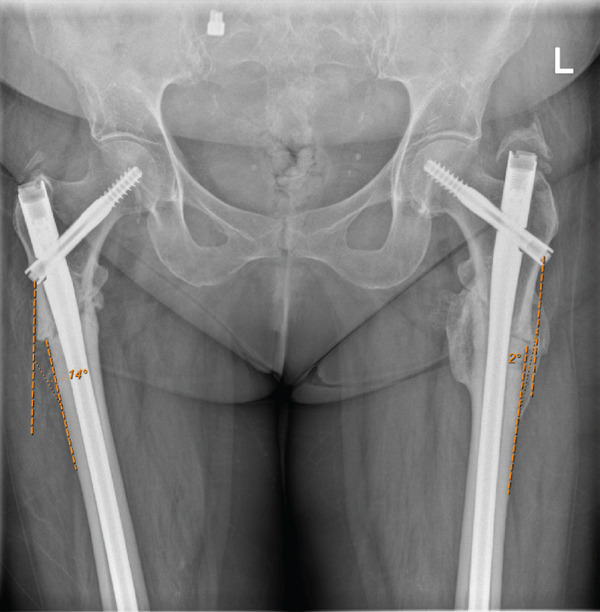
Postoperative anteroposterior radiographic imaging at 14‐month showing cephalomedullary nailings for initial bilateral femoral fracture fixation with evidence of excessive callus formation on left femur and nonunion of right femur. Noted right femur following initial fixation in varus (measured 14°).
